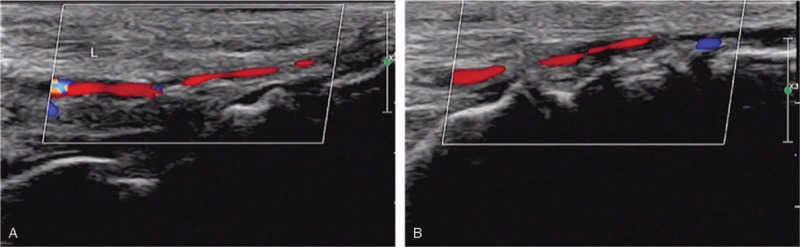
Color Doppler ultrasound images of dorsalis pedis artery. (A). Color Doppler flow image of left dorsalis pedis artery. (B). Color Doppler flow image of right dorsalis pedis artery.
